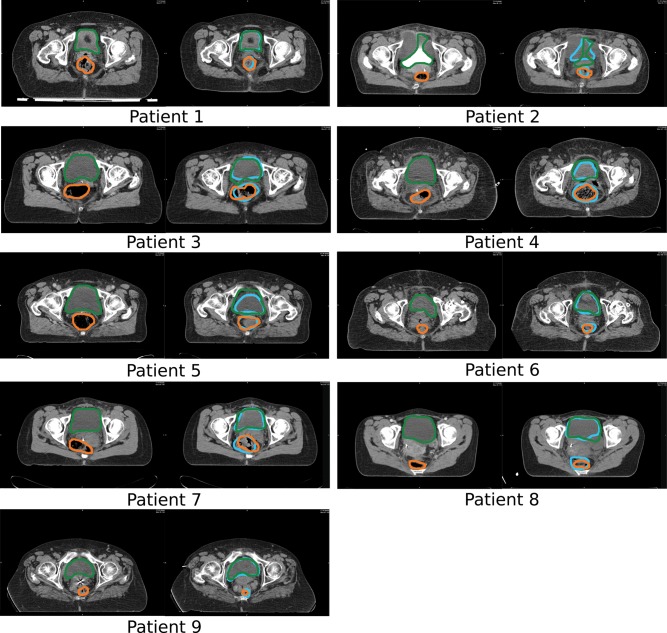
Representative axial slices from planning CT (left) and repeated CT (right) for all patients. The manually drawn bladder (green) and rectum (orange) are displayed on the planning CT. The manually contoured structures (blue) are shown in the repeated CT, together with the original planning CT structures (green and orange respectively) deformed by the DIR algorithm.
